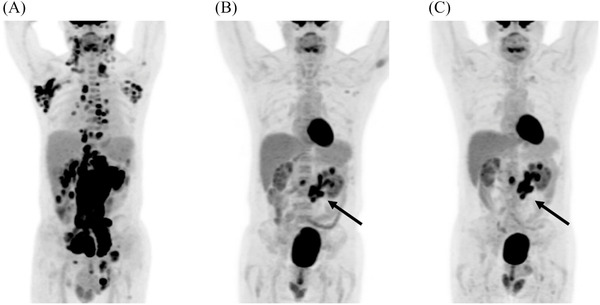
Serial select positron emission tomography‐computed tomography (PET‐CT) images during treatment: (A) before R‐CHOP chemoimmunotherapy initiation, (B) at the end of six cycles of R‐CHOP, and (C) 6 weeks after completion of R‐CHOP
